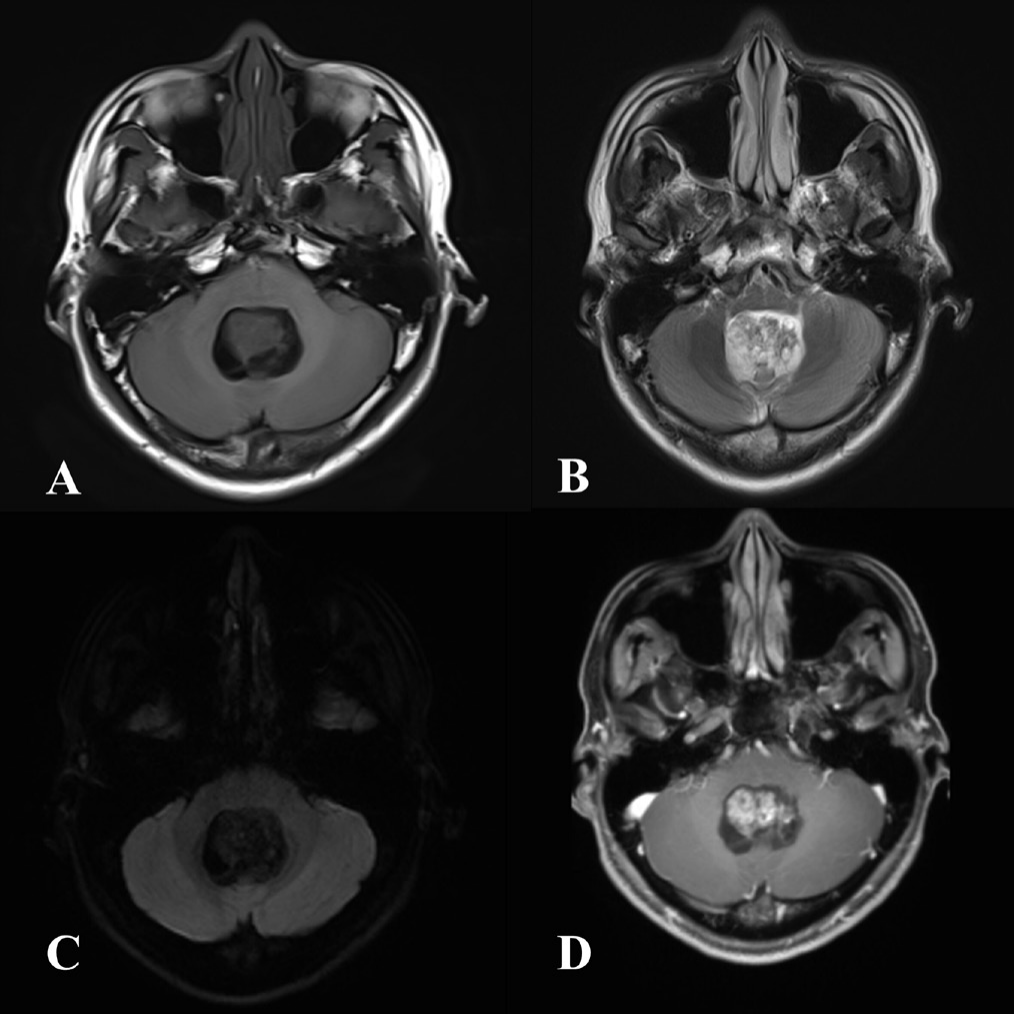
A 27-year-old male patient with intermittent posterior occipital distension and heaviness. MR examination: A. isohypointensity on axial *T*_1_ weighted imaging; B. isohyperintensity on axial *T*_2_ weighted imaging; C. the lesion appears hypointense on DWI; D. heterogeneous enhancement on the *T*_1_ weighted image with gadolinium. DWI, diffusion-weighted imaging.
